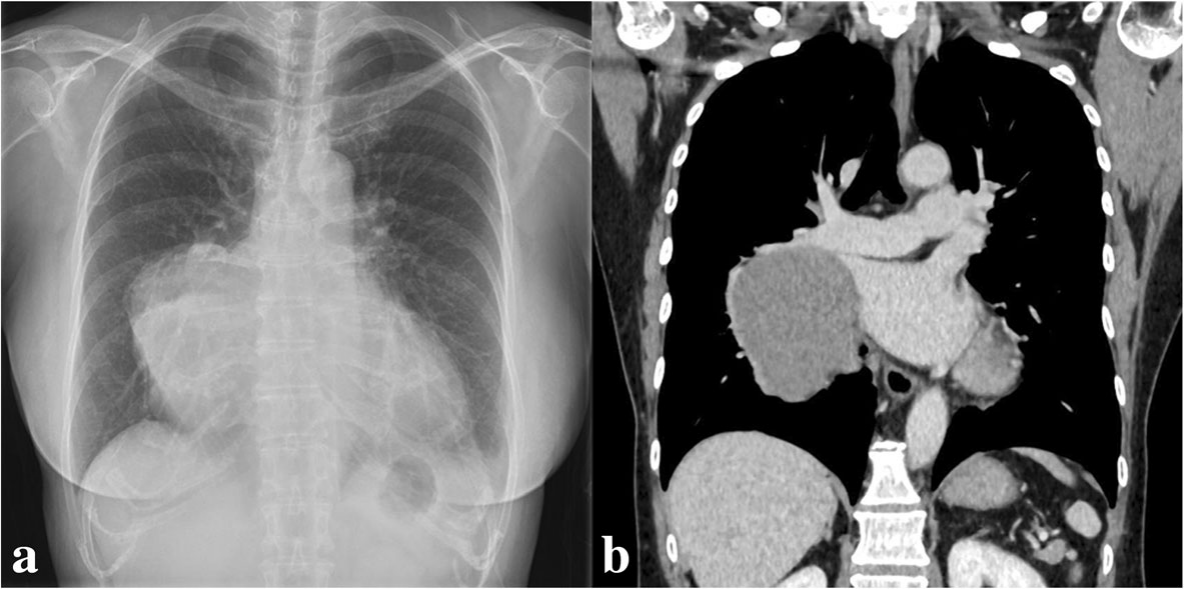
Chest radiography (a) and chest computed tomography (b) at the first visit show a large mass in the right lower lung field
